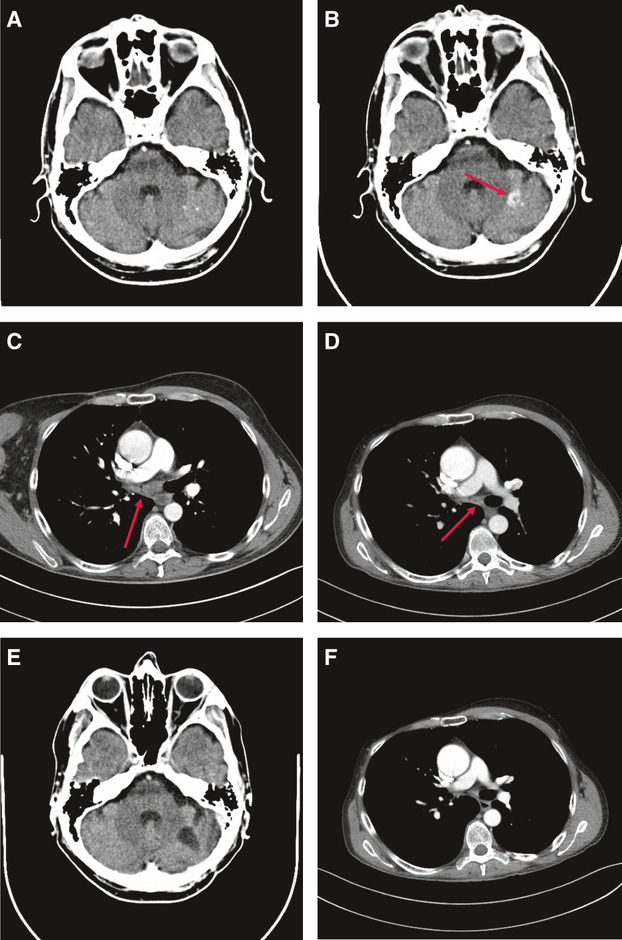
Computed tomography (CT) scans before and during treatment with vemurafenib (patient 1). (A) CT scan (August 2010) before treatment: no cerebral metastases. (B) CT scan (May 2011) during treatment: new cerebral metastasis. (C) CT scan (August 2010) before treatment: hilar lymph node metastases. (D) CT scan (May 2011) during treatment: regression. (E) CT scan (August 2012) during treatment: complete remission of extracerebral metastases. (F) CT scan (August 2012) during treatment: no cerebral metastases.
